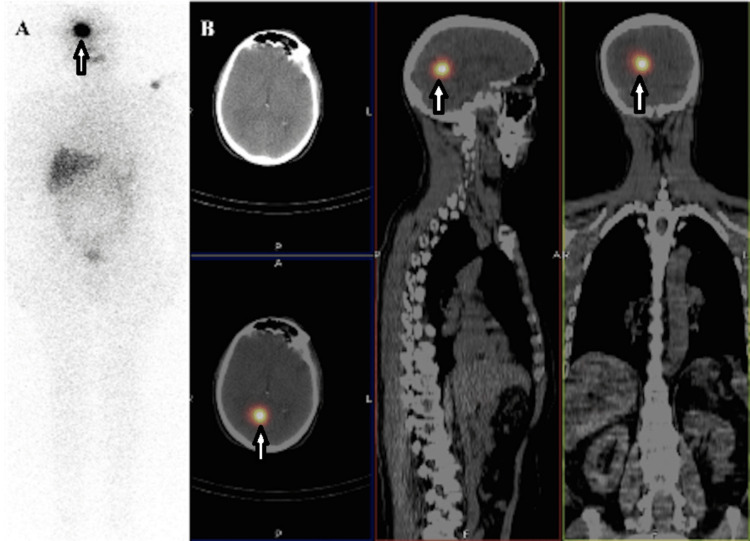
(A) Whole-body post-therapeutic I-131 scan demonstrating focal intense iodine uptake in the right frontal region of the cranium and the left shoulder (white arrows). (B) I-131 SPECT/CT fusion images confirming focal intense iodine uptake in the right occipital lobe (white arrows). The I-131 uptake on the left shoulder was evaluated as external contamination.
